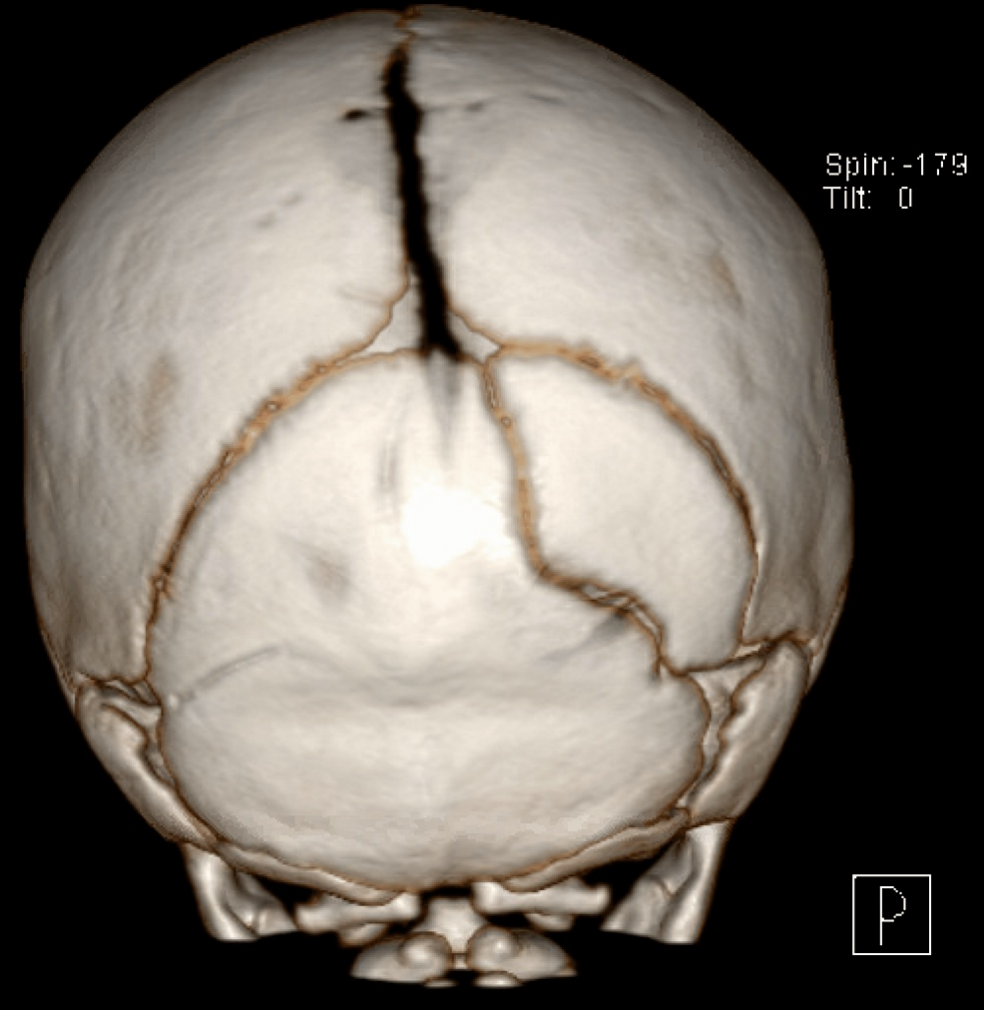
3D CT scan findings of accessory cranial sutures in a two-month-old male coronal view. The figure shows an extraneous suture from an undetermined cause.
